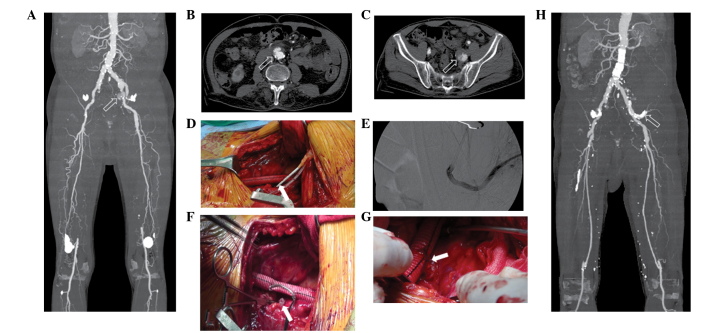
Case 2. A 69-year-old male patient had (A and C) a left internal iliac artery (IIA) aneurysm (open arrows), (A) bilateral superficial femoral artery occlusion and (B) focal aneurysmal change of the infrarenal abdominal aorta (open arrow). (D and E) Endovascular repair of the IIA aneurysm was performed with direct puncture of the aneurysm (white arrow). (F and G) A covered stent to repair the IIA aneurysm was anastomosed with the iliac bypass graft (white arrows). (H) Postoperative computed tomography-angiography showed that there were no endoleaks and that the covered stent graft to repair the IIA aneurysm was patent (open arrow).
